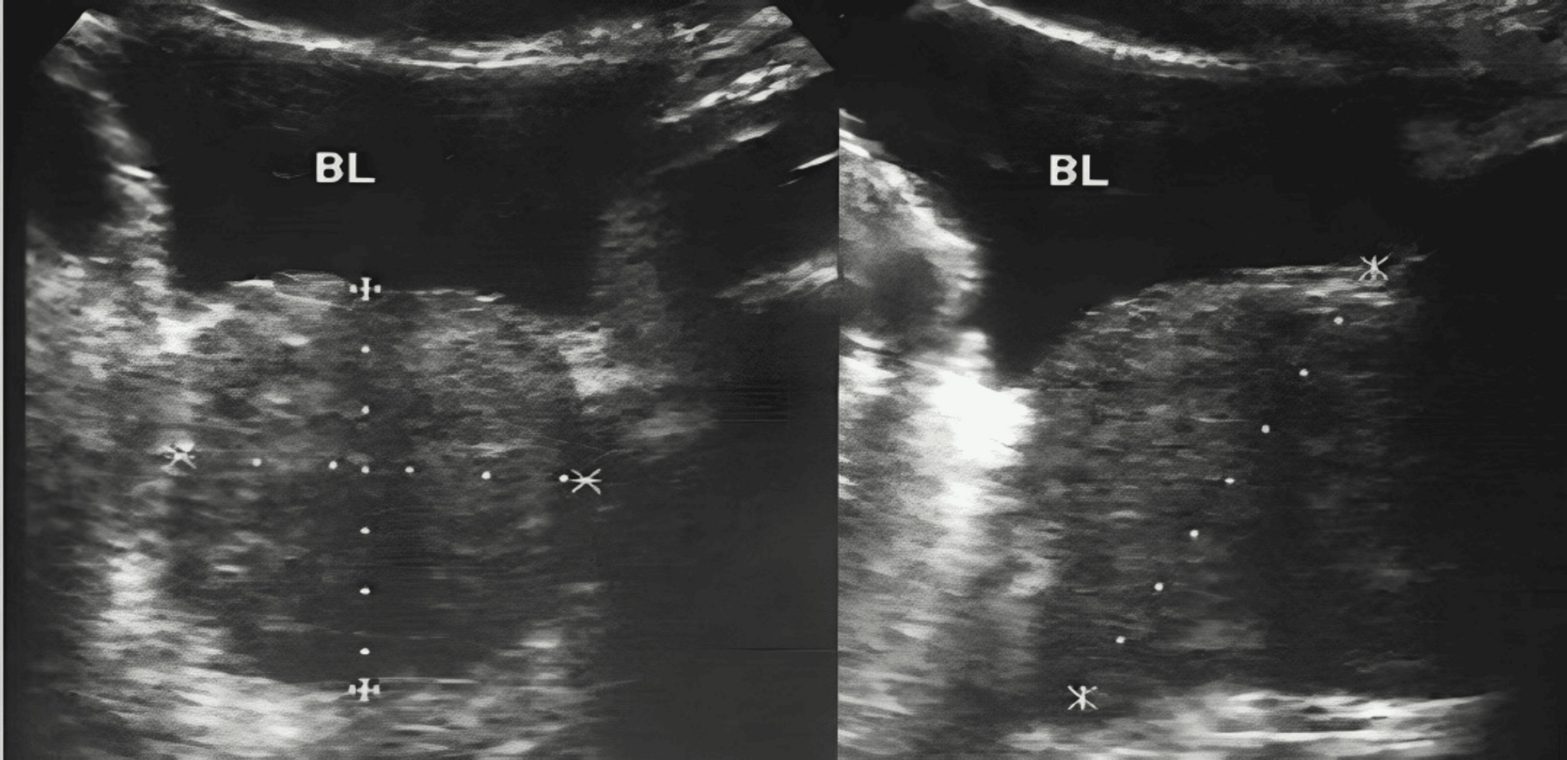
Ultrasound of the kidneys, ureters, and bladder (USG KUB) showing Grade III prostatomegaly with a homogeneous echotexture. BL: bladder.
